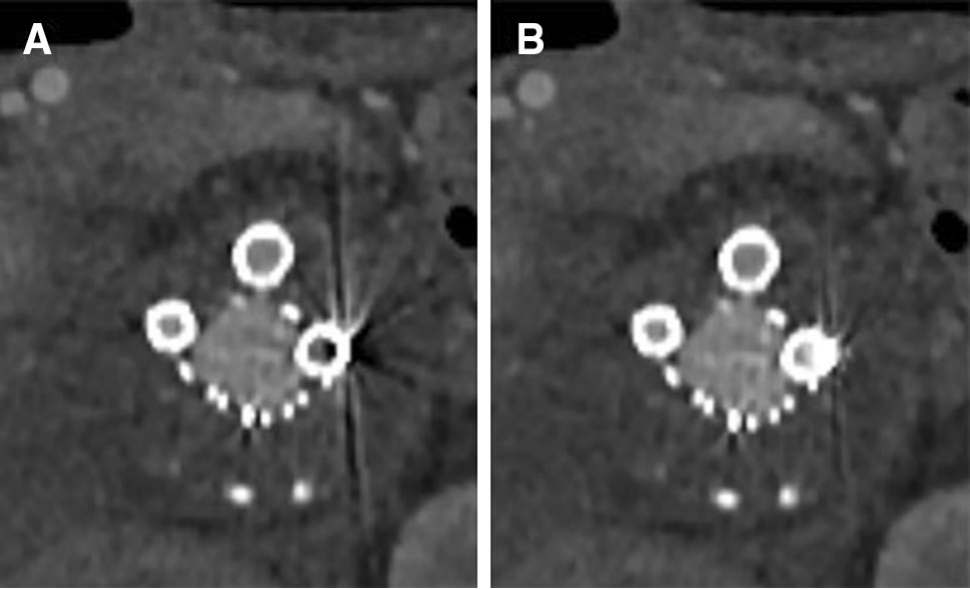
CT images (A standard (AIDR 3DE), B SEMAR) of a branch connected to the superior mesenteric artery and two branches connected to the renal arteries in a 66-year-old male. Notice the decrease in streaking artifacts in the left renal artery. Although artifacts are reduced significantly, the visualization of stent patency is still limited
